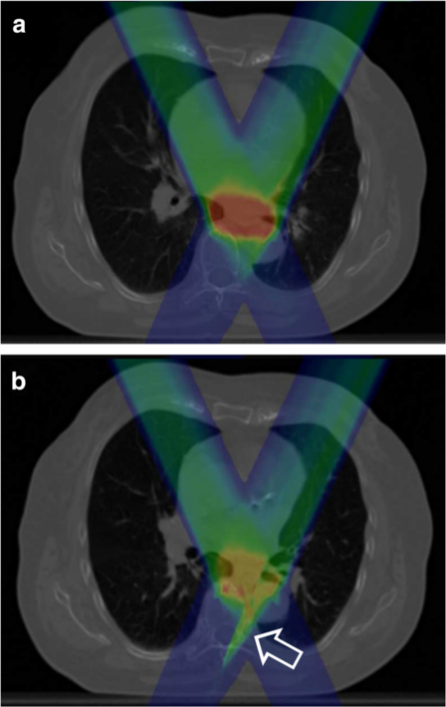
Dose distribution for patient 3 with geo8 calculated a) on the free-breathing planning CT and b) on the 4D-CT phase Ex0% with a critical overshoot due to slight variations of the left heart contour (*arrow*)
